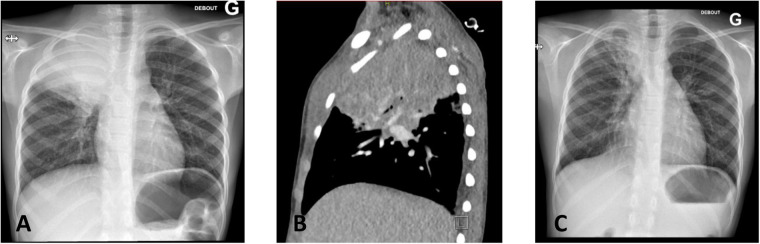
Radiological findings of our patient: (A) first X-ray showing consolidation of the right upper lobe – (B) computed tomography before treatment showing consolidation of the right upper lobe with extension in the anterior chest wall – (C) X-ray after treatment completion showing complete regression of the consolidation.
